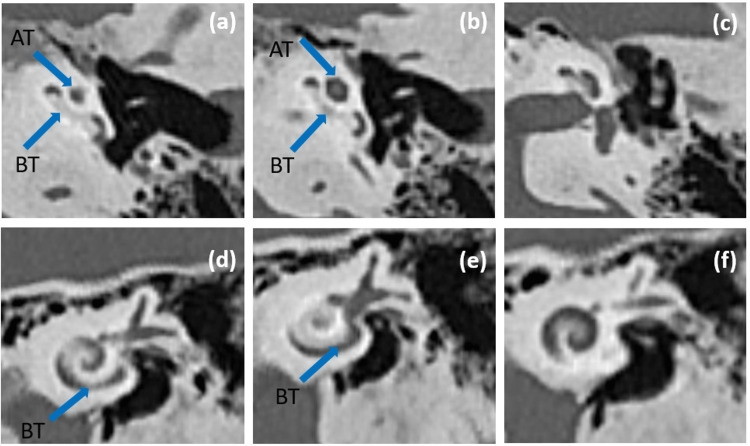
(a-c): axial CT scan of the left cochlea, and (d-f): coronal CT scan of the left cochlea. Both views revealed the cochlea with relatively confluent hyperdense plaque of mineralization in the basal and middle turns just proximal to the round window. AT: apical turn. BT: basal turn.
